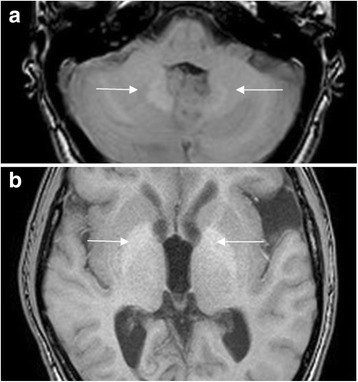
Unenhanced T1-w MRI of a VHL patient, showing spontaneous hypersignal (arrows) in dentate nucleus (a) en globus pallidus (b)
